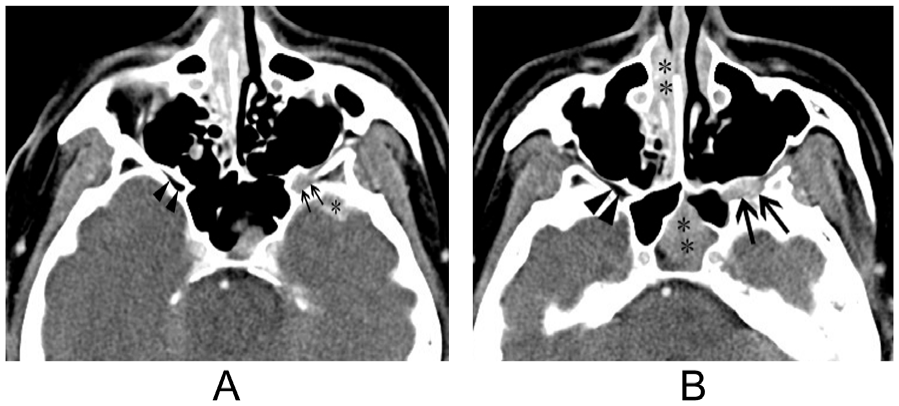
Idiopathic orbital inflammation syndrome with pterygopalatine fossa and middle cranial fossa involvement. Figure 2A: Axial contrast-enhanced CT imaging reveals enlargement of and abnormal soft tissue within the left inferior orbital fissure (arrows). Compare this to the normal inferior orbital fissure on the contralateral side (arrowheads). Note the involvement of the left middle cranial fossa (asterisk). Figure 2B: Axial contrast-enhanced CT imaging reveals enlargement of and abnormal soft tissue within the left pterygopalatine fossa (arrows). Compare this to the normal pterygopalatine fossa on the contralateral side (arrowheads). Note the sphenoidal and right ethmoidal sinusitis (asterisks).
